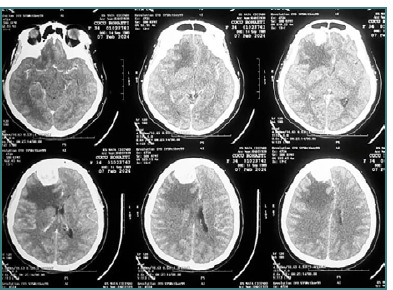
CT scan shows compressed sulcus and gyrus, compressed Sylvian fissure, peritumoral edema, and midline shift
